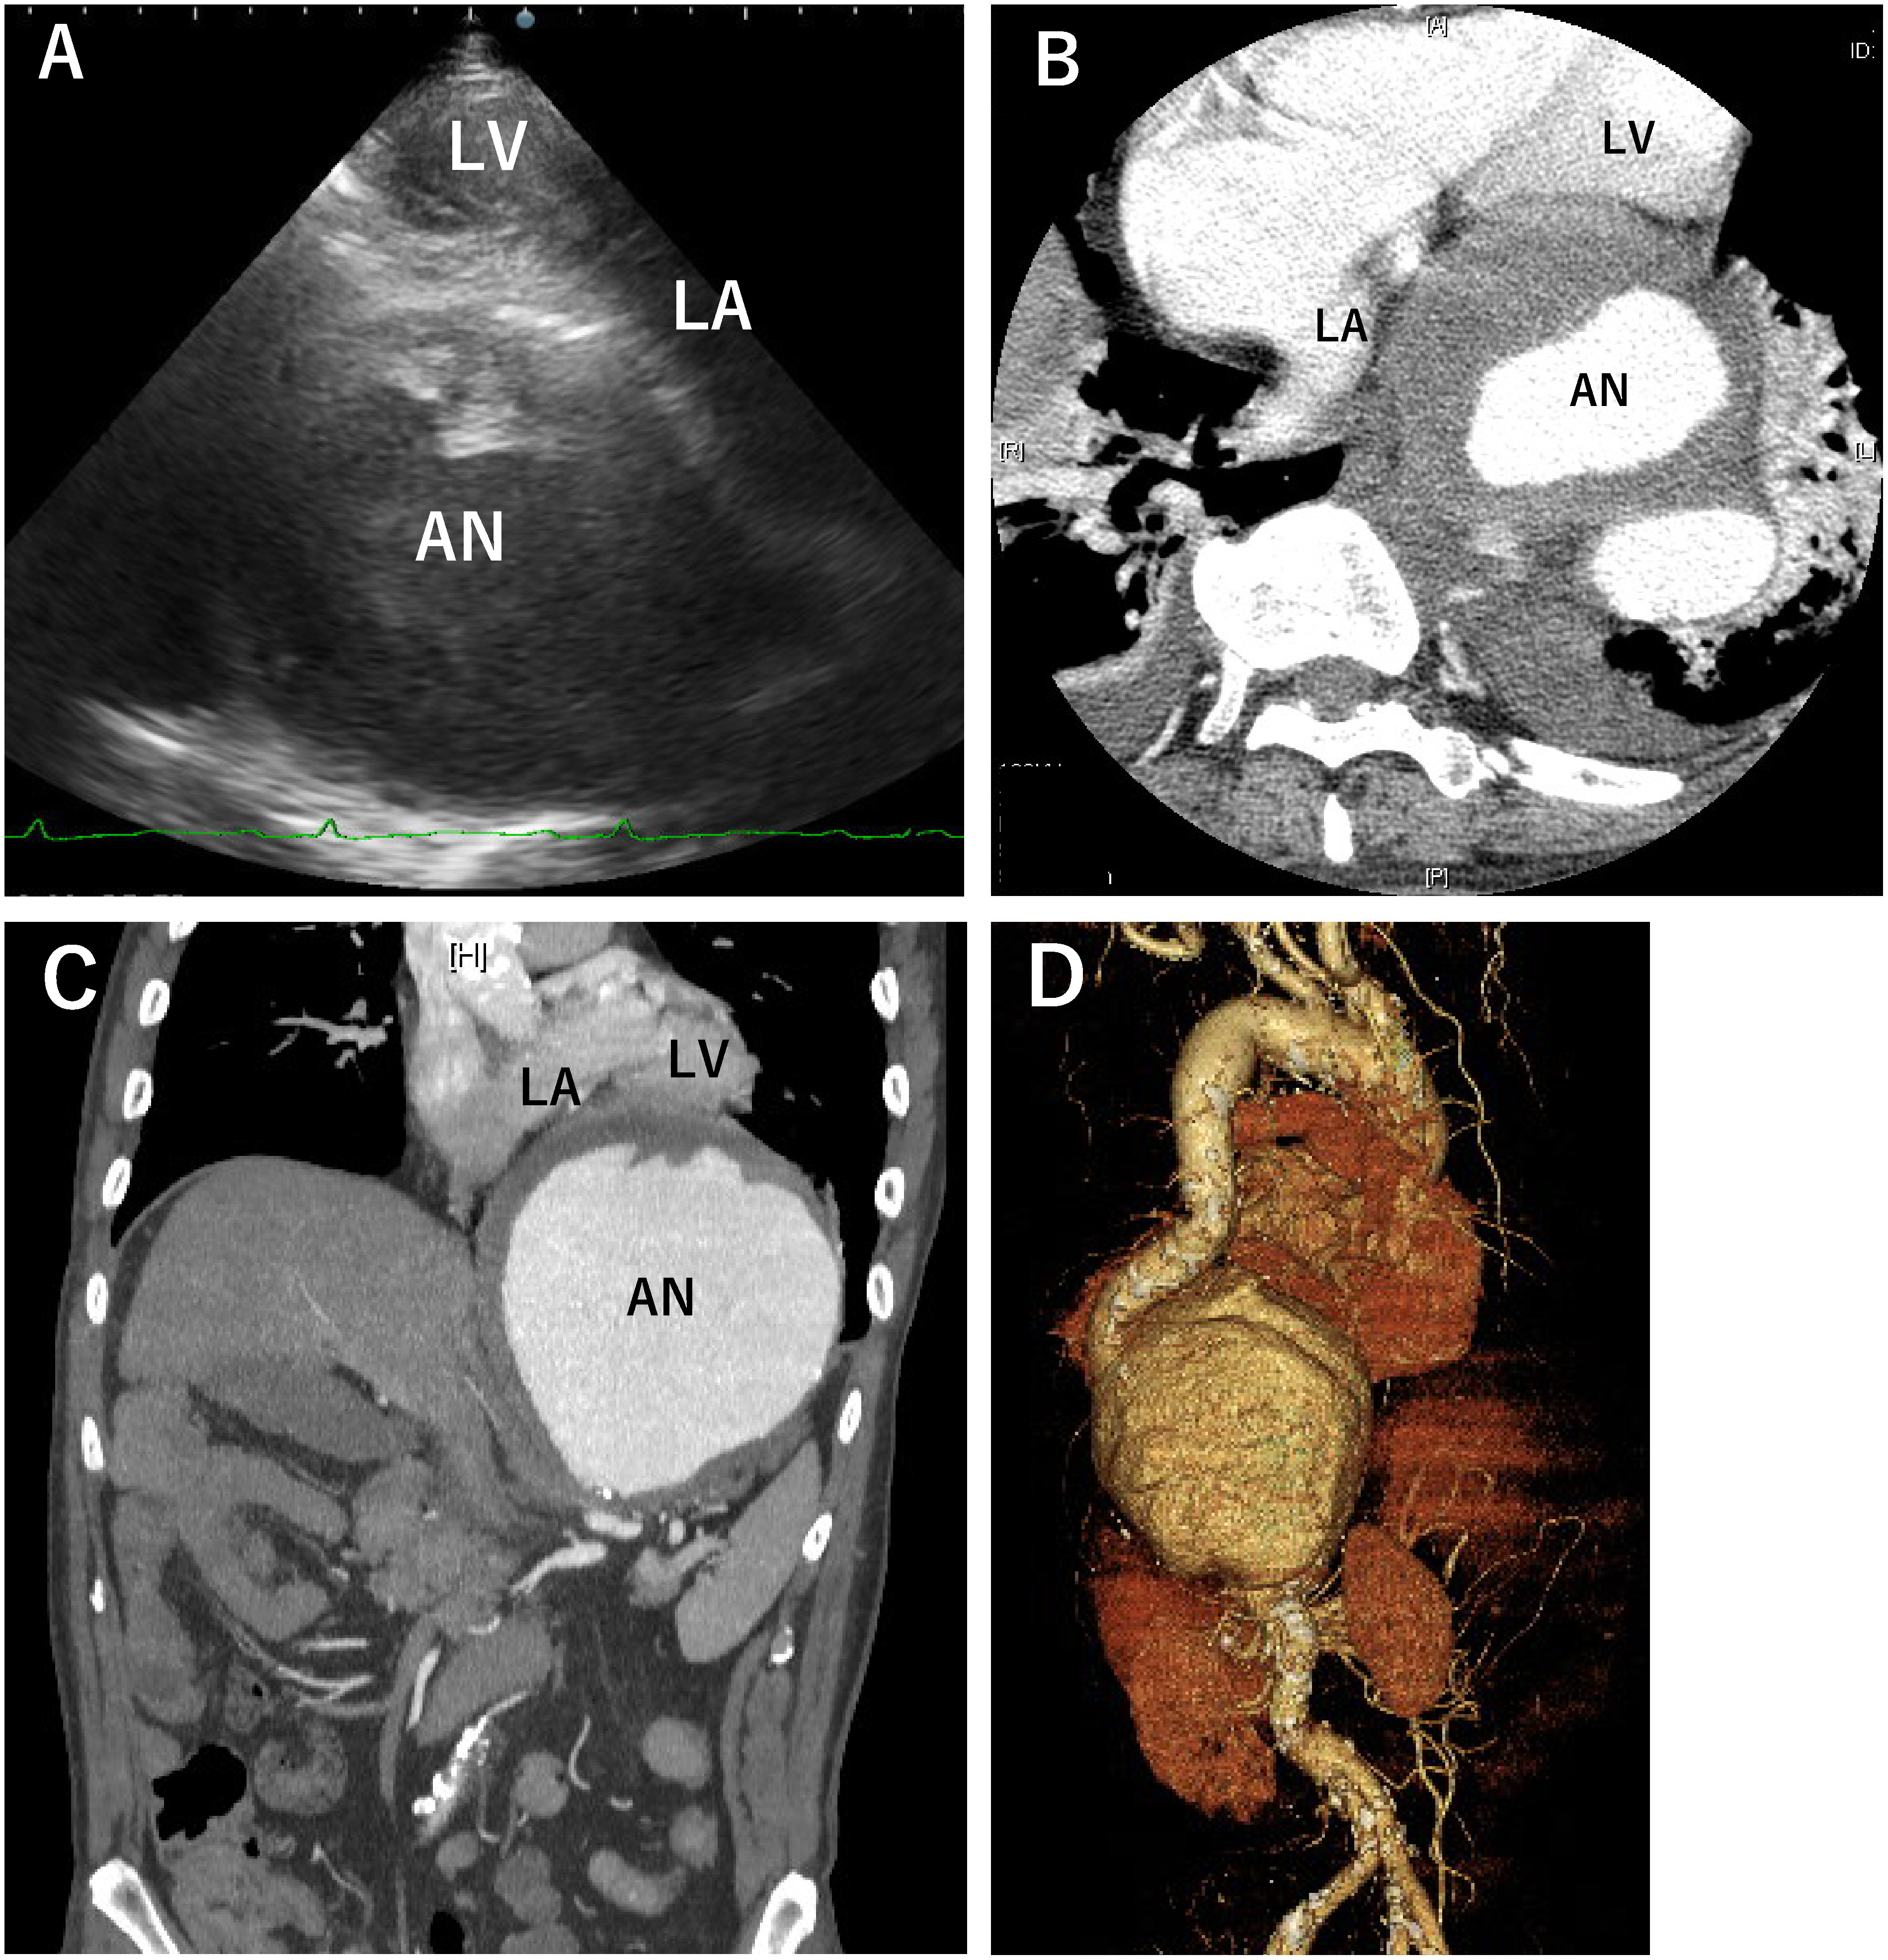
Fig. 1 Preoperative transthoracic echocardiography (A) and contrast-enhanced computed tomography in the axial (B) and coronal (C) planes showing compression of the LA and LV by a thoracoabdominal aortic aneurysm. Three-dimensional computed tomography image (D) showing a giant aneurysm in the thoracoabdominal aorta measuring 140 mm in diameter.
